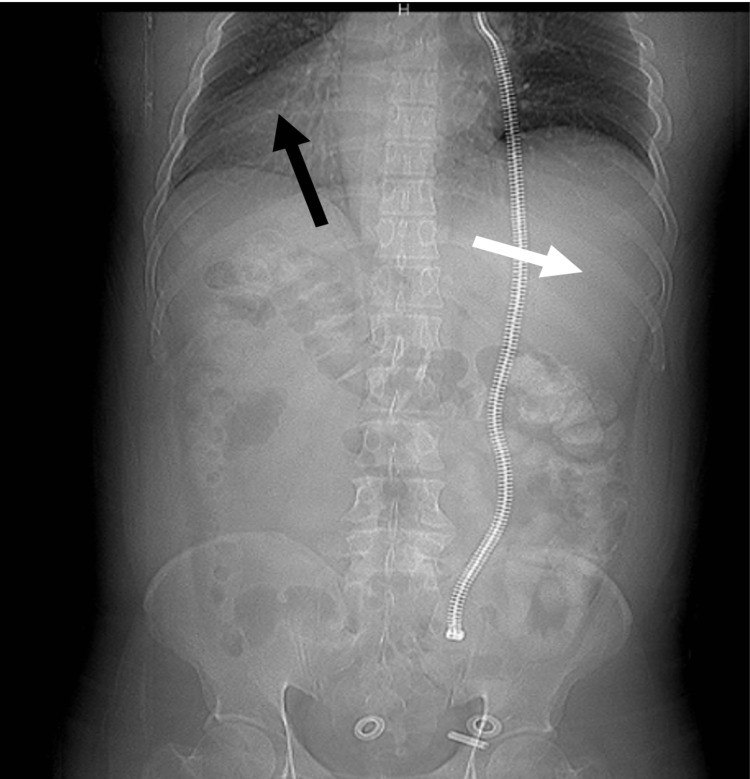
Chest radiograph showing situs inversus. A cardiac silhouette was observed in the right hemithorax (black arrow). A hepatic silhouette was observed in the left hemiabdomen (white arrow).
